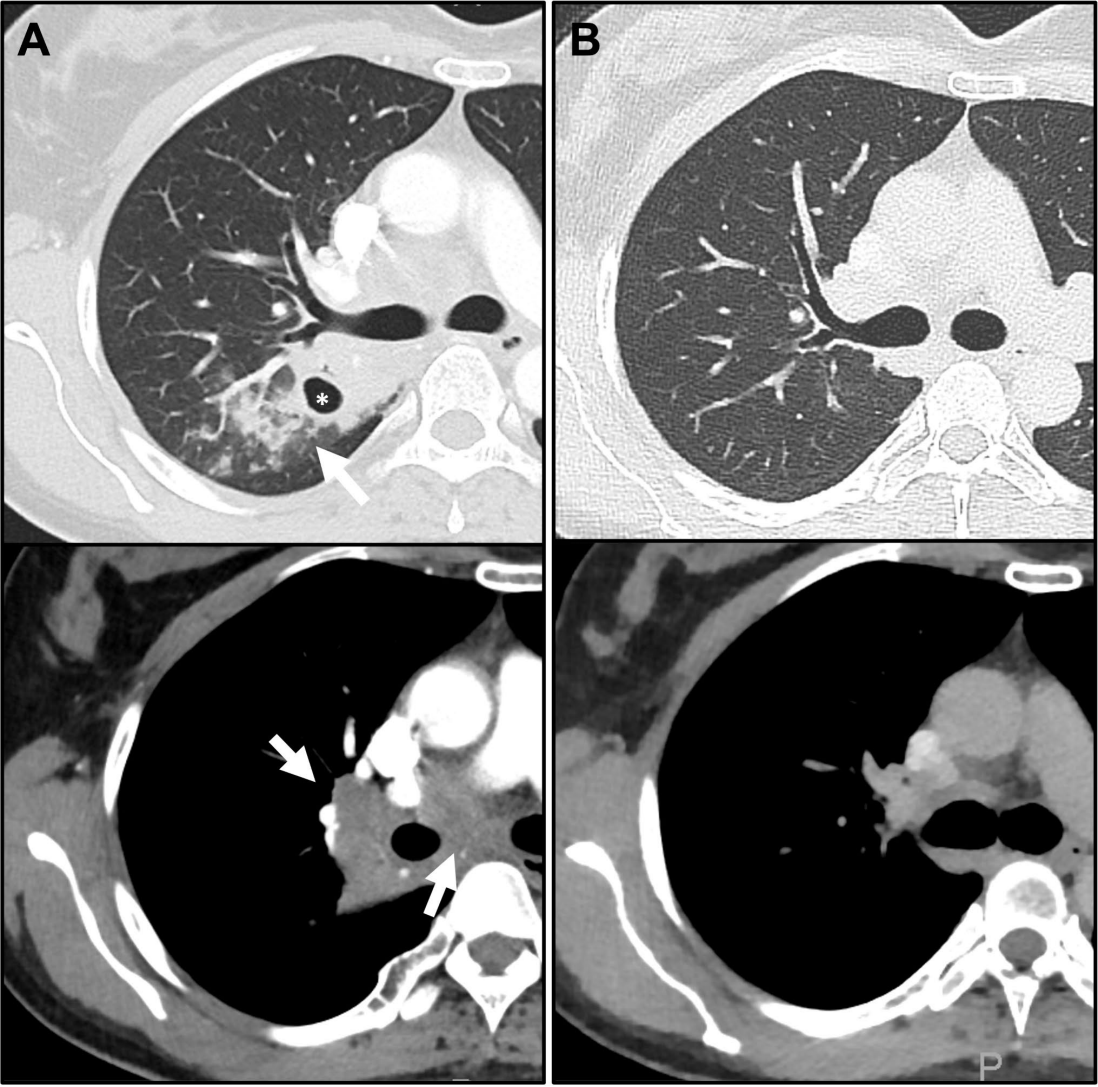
Computed tomography at baseline (A) and follow-up (B) in lung window (upper images) and soft-tissue window (lower images). A shows pulmonary infiltration in the right upper lobe with predominance of bronchocentric consolidations (upper image, arrow) and evidence of intralesional pulmonary cavitation (upper image, asterisk). There is coalescing adenopathy at the right hilum and infracarinary mediastinum (lower image, arrows). Follow-up imaging after 8 months (B) shows complete resolution of pulmonary infiltrates and adenopathy. (courtesy of Mathias Beyer, MD, Radiologie Lampertheim for A)
